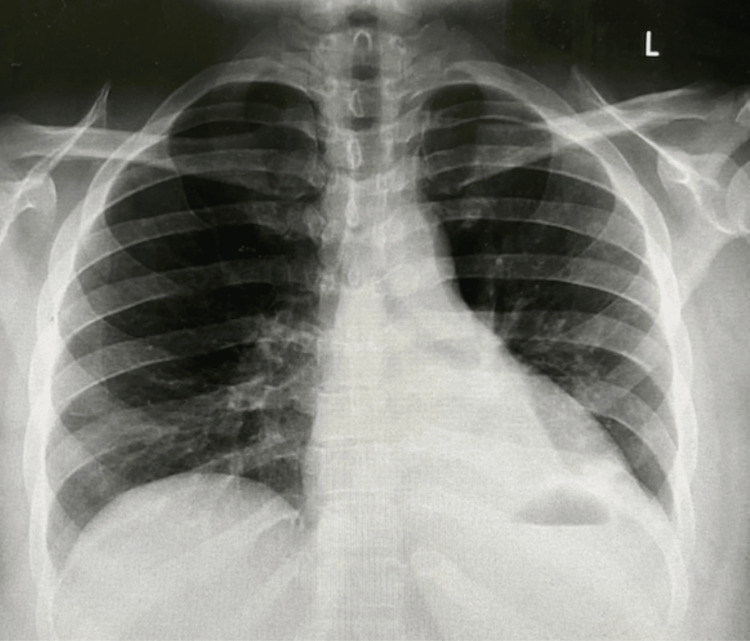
Chest X-ray after bronchoscopy six days after admission
